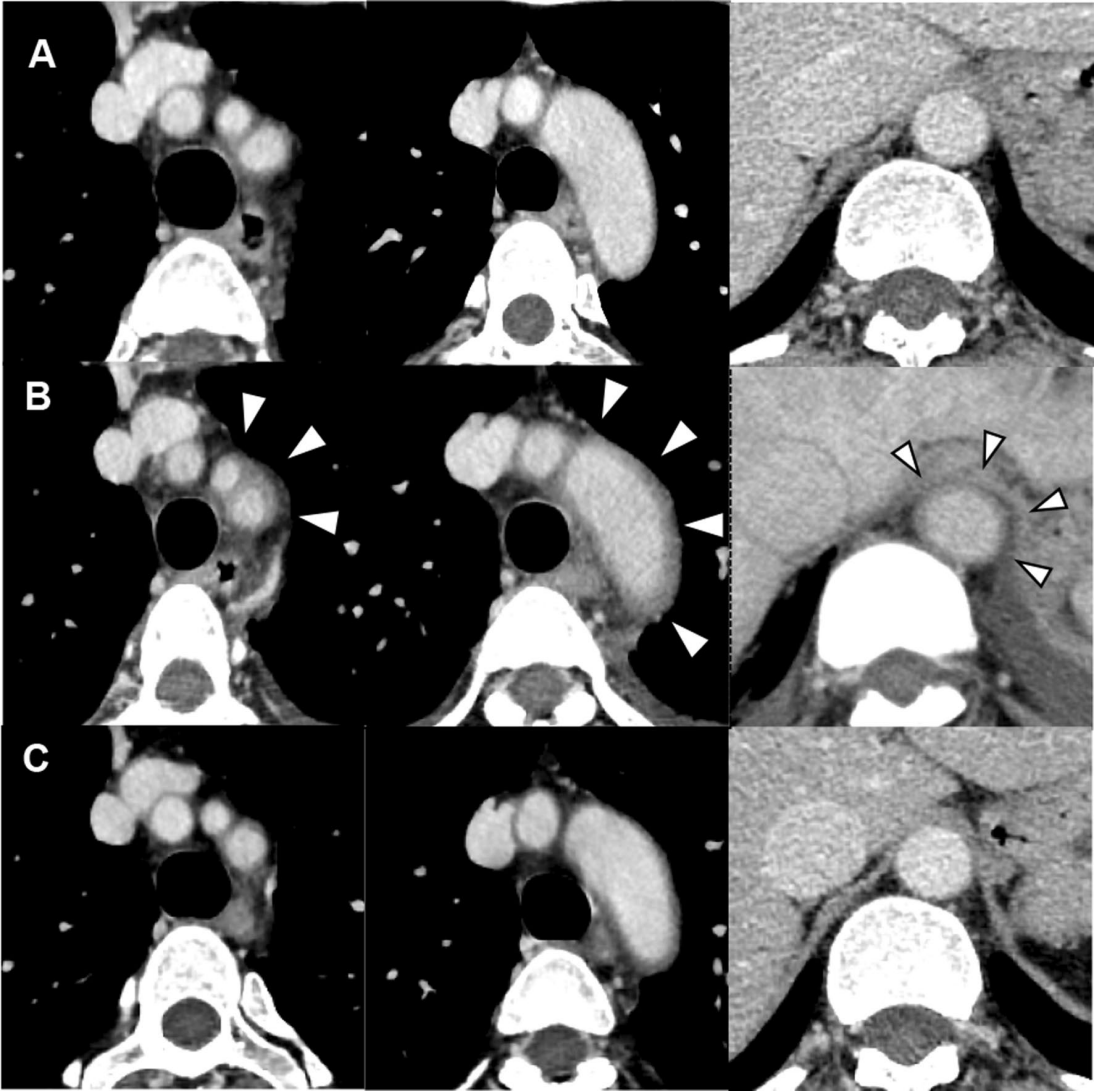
Contrast-enhanced CT images before and after treatment. A CT images before chemotherapy. B Contrast-enhanced CT images before treatment (day 8), showing thickened vessel walls in the left subclavian artery, the origin of the common carotid artery, and the thoracoabdominal aorta (white arrowheads). C Contrast CT images after treatment (day 22), showing improvement in the perivascular wall thickening. *CT* computed tomography
